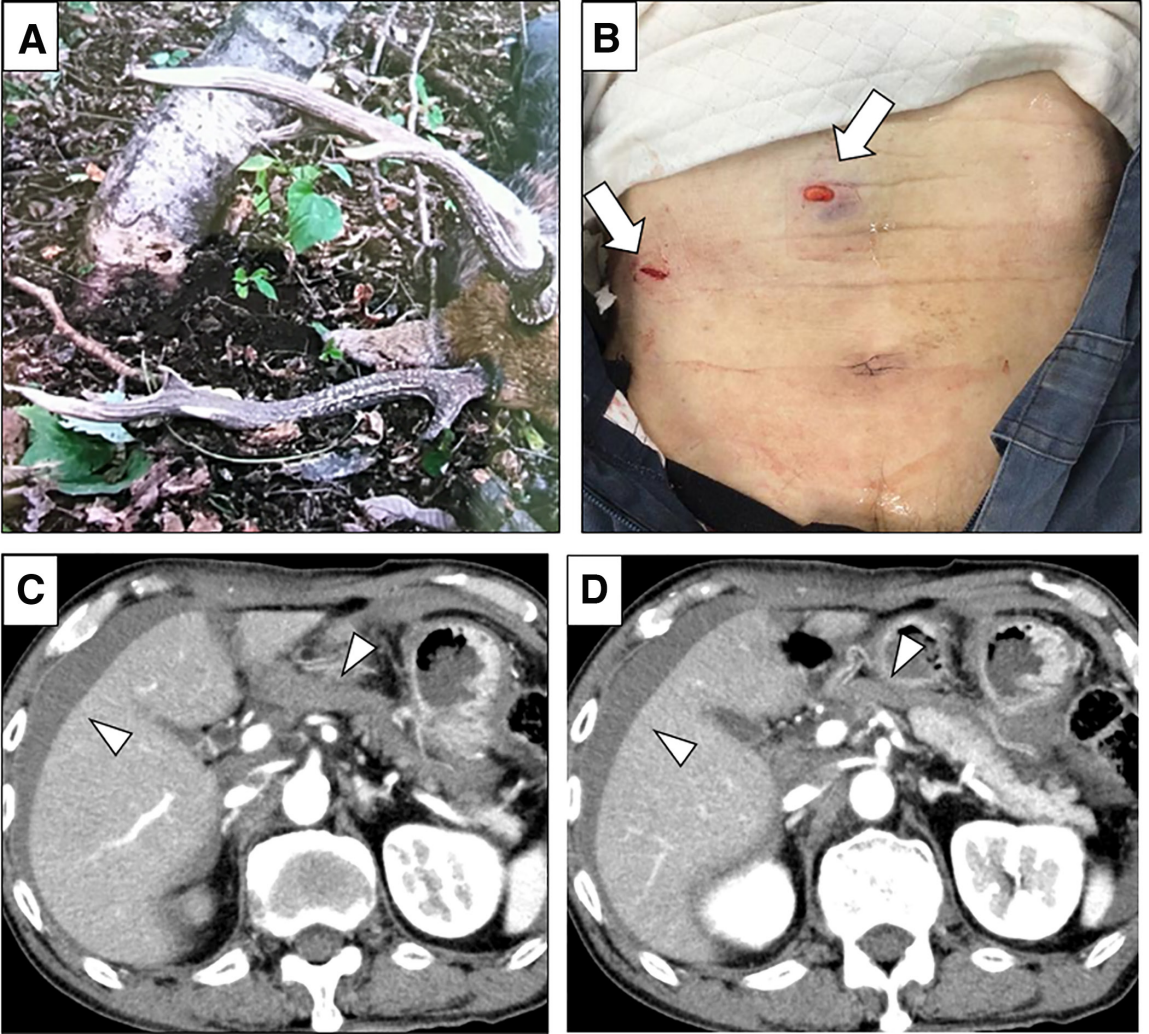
Physical examination findings and preoperative CT. (A) The deer antlers that caused the injury were approximately 40 cm in length. (B) Two abdominal wall wounds (arrows). (C and D) Contrast-enhanced CT revealed a large amount of intraperitoneal fluid (arrowheads), with no evidence of active extravasation, free air, or solid organ injury.
